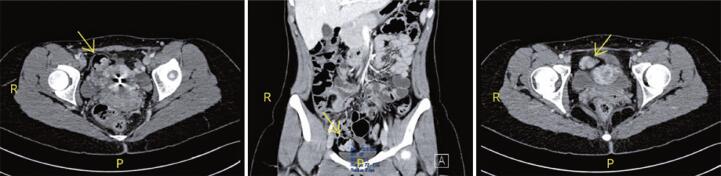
Examination images - computed tomography, portal phase, performed as an emergency, without oral contrast. Yellow arrows point to a blind-end loop in the right iliac fossa, raising a diagnostic hypothesis of Meckel’s diverticulum
